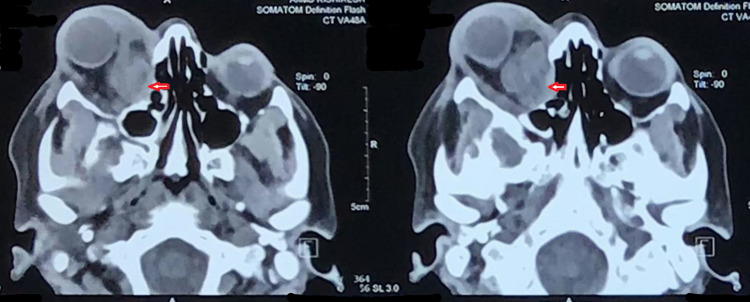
CECT of orbits and paranasal sinuses showing a retrobulbar mass with a dense calcific focus, extending to intraconal and extraconal spaces (red arrows). CECT: Contrast-enhanced computed tomography.
